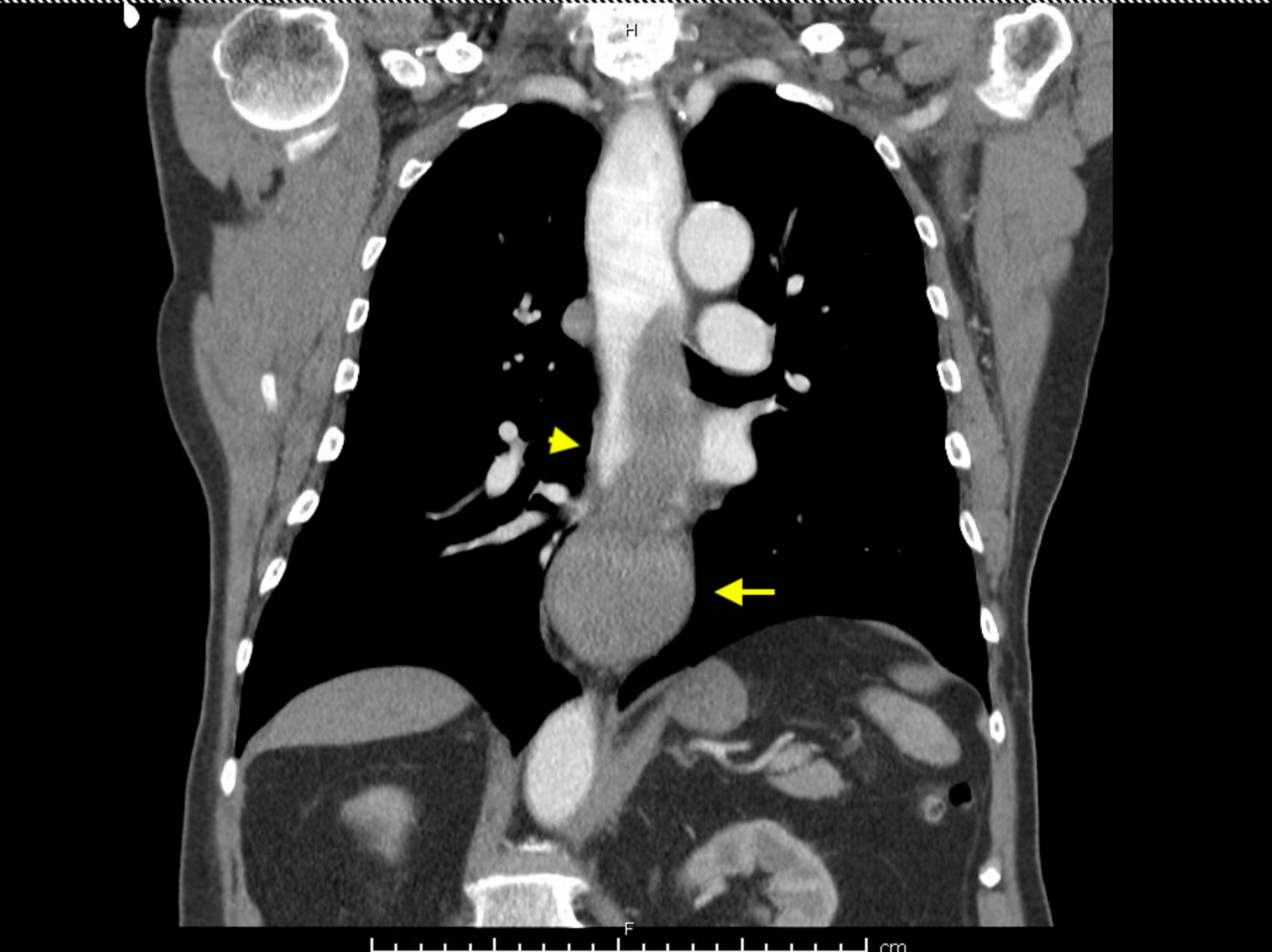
Computed tomography (CT) thorax with oral and intravenous (IV) contrast showing esophageal luminal narrowing at the distal third (yellow arrowhead) with mural thickening and a soft tissue density extending to the gastroesophageal junction (yellow arrow)
